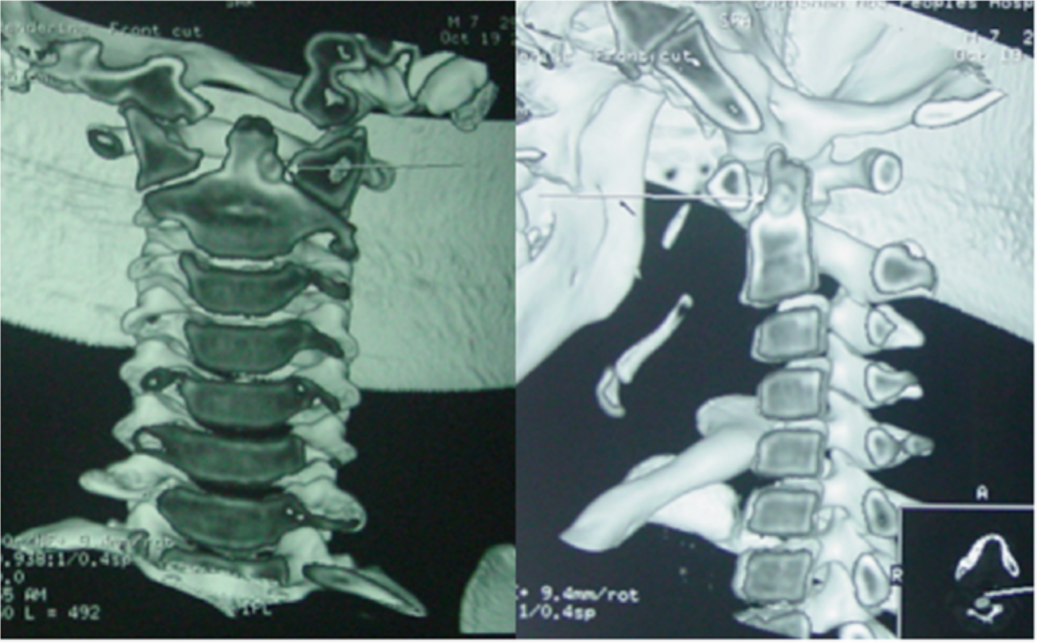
Computed tomography (CT) of three-dimensional (3-D) reconstruction of the nidus.
